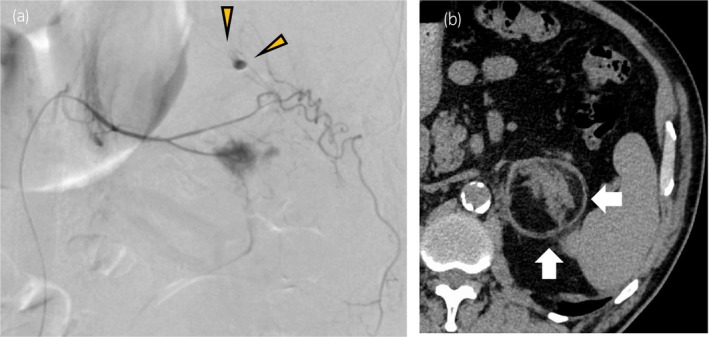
Angiogram and computed tomography radiographs of the patient after catheterization. (a) Selective catheterization and angiogram of the left middle adrenal artery showing extravasation of contrast medium (yellow arrowheads). (b) Four months after embolization, an abdominal CT scan shows a left AML of 58 × 57 mm^2^ (white arrow).
